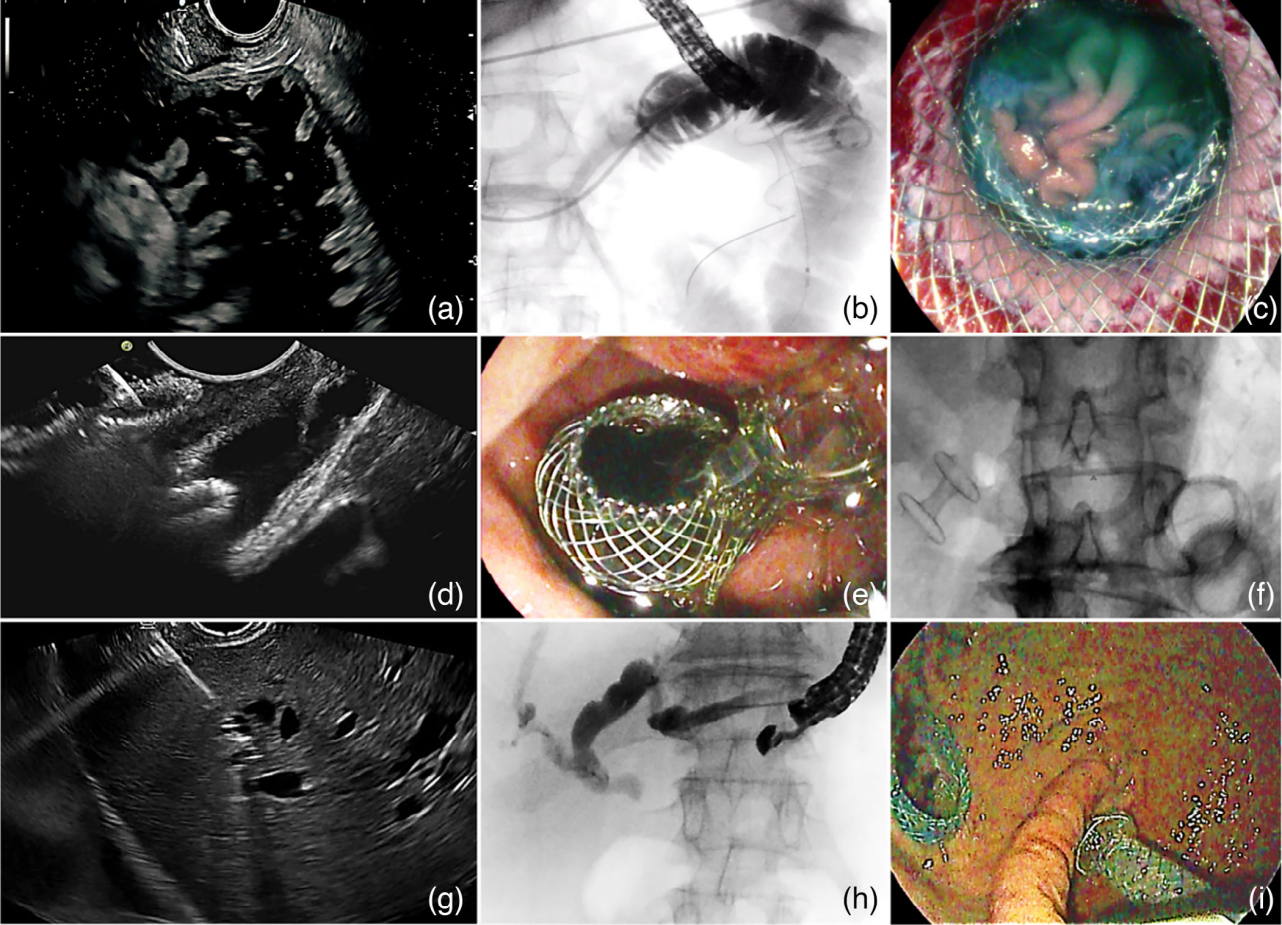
(a–c) Endoscopic ultrasound (EUS)‐guided gastroenterostomy (EUS‐GE). (a) Endosonographic appearance of the operative window for EUS‐GE before lumen‐apposing metal stents (LAMS) deployment (see the tip of the catheter in the left upper corner). The operative space includes an adequately distended jejunal loop, orientated in the direction of the operative channel of the scope, with the shortest distance possible between the gastric and jejunal lumen; (b) fluoroscopic image showing an oro‐jejunal tube looped in the first jejunal loop, through which the jejunum is distended (in this case with saline mixed with contrast) and a LAMS released between the stomach and the jejunal loop. (c) Visualization of the jejunal folds through the LAMS after stent release and dilation. (d–f) EUS‐guided choledochoduodenostomy (EUS‐CDS). (d) Endosonographic appearance of a LAMS placed between the choledochus and the duodenal bulb; (e) endoscopic image of a released proximal flange with bile flowing in the duodenum; (f) fluoroscopic image of a double obstruction managed by EUS‐GE + EUS‐CDS (with aerobilia). (g–i) EUS‐guided hepaticogastrostomy. (g) Endosonographic appearance of a 19G needle accessing a branch of a mildly dilated left biliary three through a cushion of hepatic parenchyma; (h) contrast injection confirming the puncture of a branch of the left biliary tree; (i) endoscopic appearance of a gastroenteric LAMS and of a hepatogastric SEMS.
